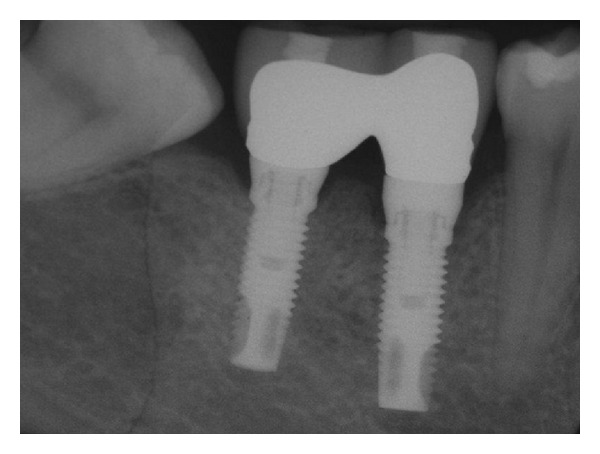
Periapical radiograph of the implants 8 years after implant placement. Substantial stability of peri-implant bone levels can be appreciated.
